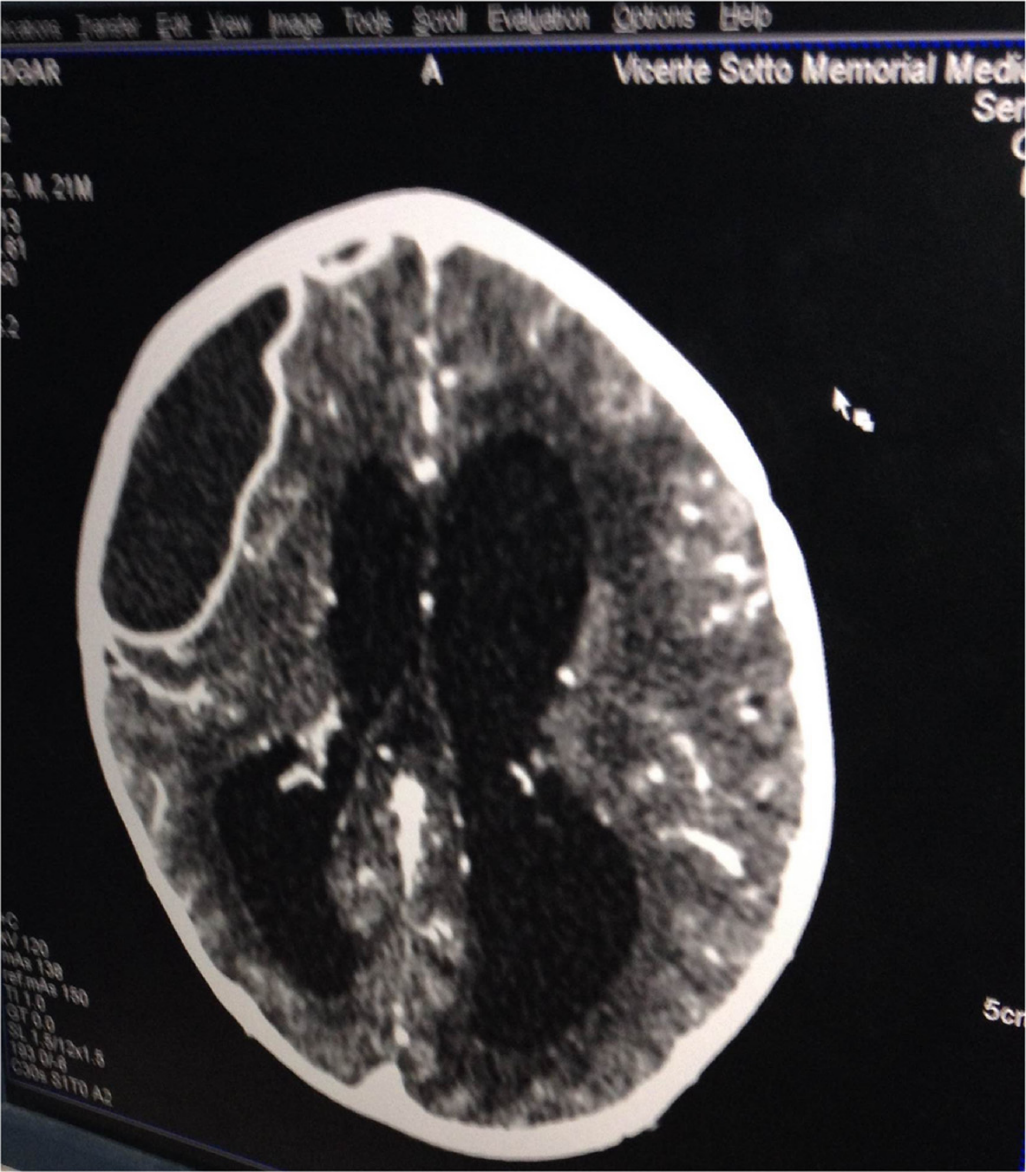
A picture (taken with a smartphone) of the child’s Brain CT showing an obvious cystic mass in the right fronto-temporal region and seemingly enlarged ventricles.
